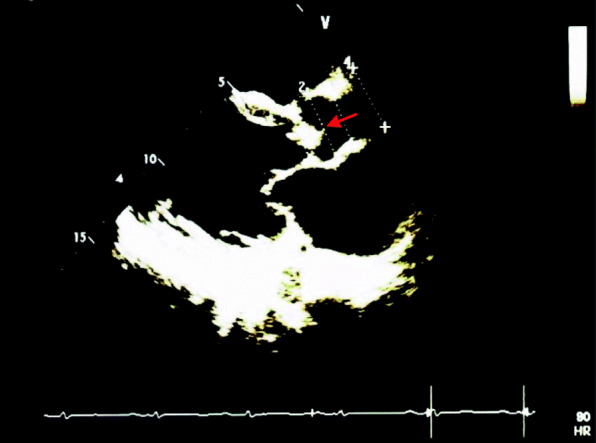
Transthoracic echocardiography shows thickened aortic leaftlets with vegetations attached to aortic cusps (red arrow)
